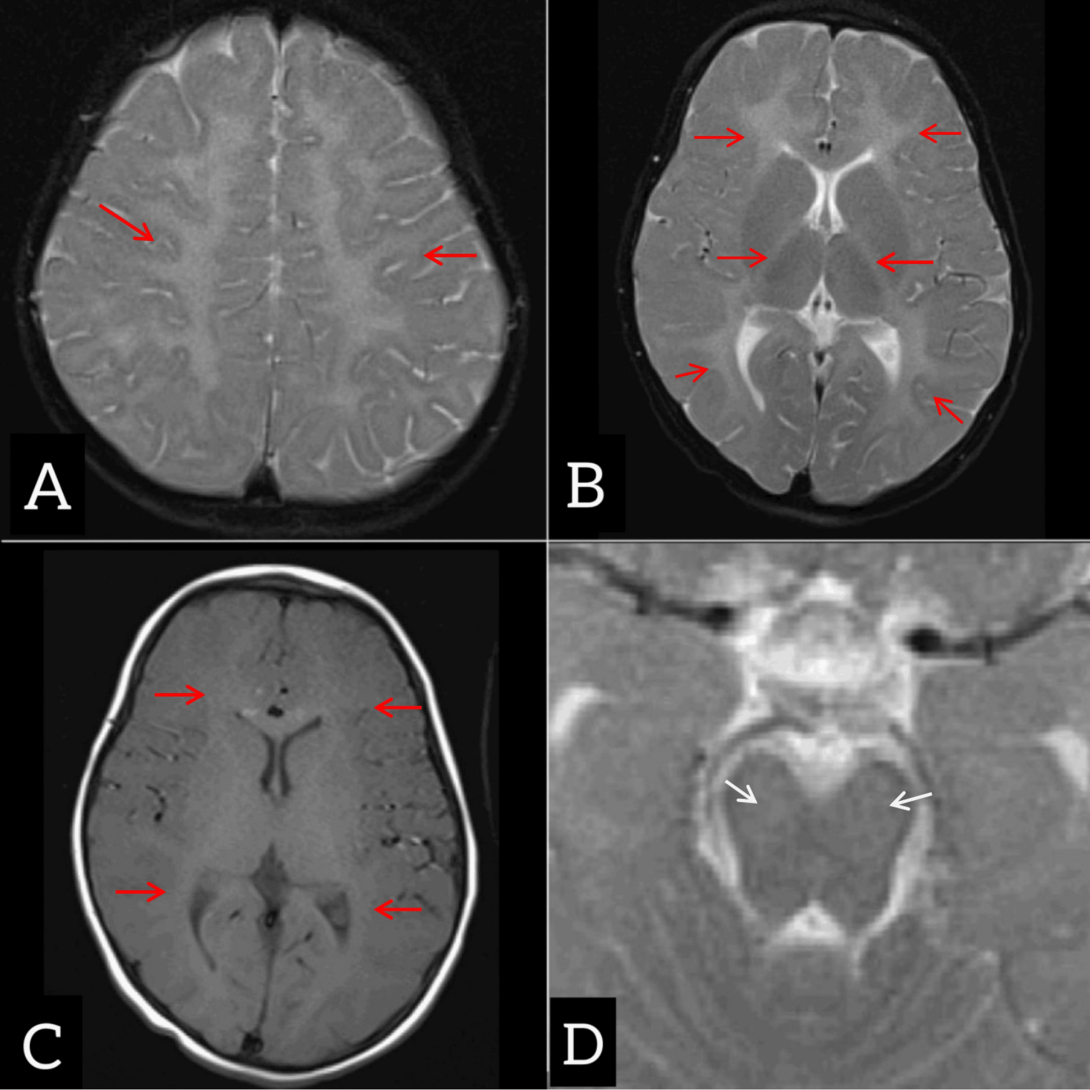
Axial sections of T2-weighted (A and B) and T1-weighted (non-contrast) (C) images showing diffuse hypomyelination of the white matter of bilateral cerebral hemispheres, including the subcortical and deep white matter and bilateral internal capsule (red arrows). Axial sections of T2 WI (D) at the level of the midbrain show patchy, subtle hypomyelination involving the midbrain (white arrows)
